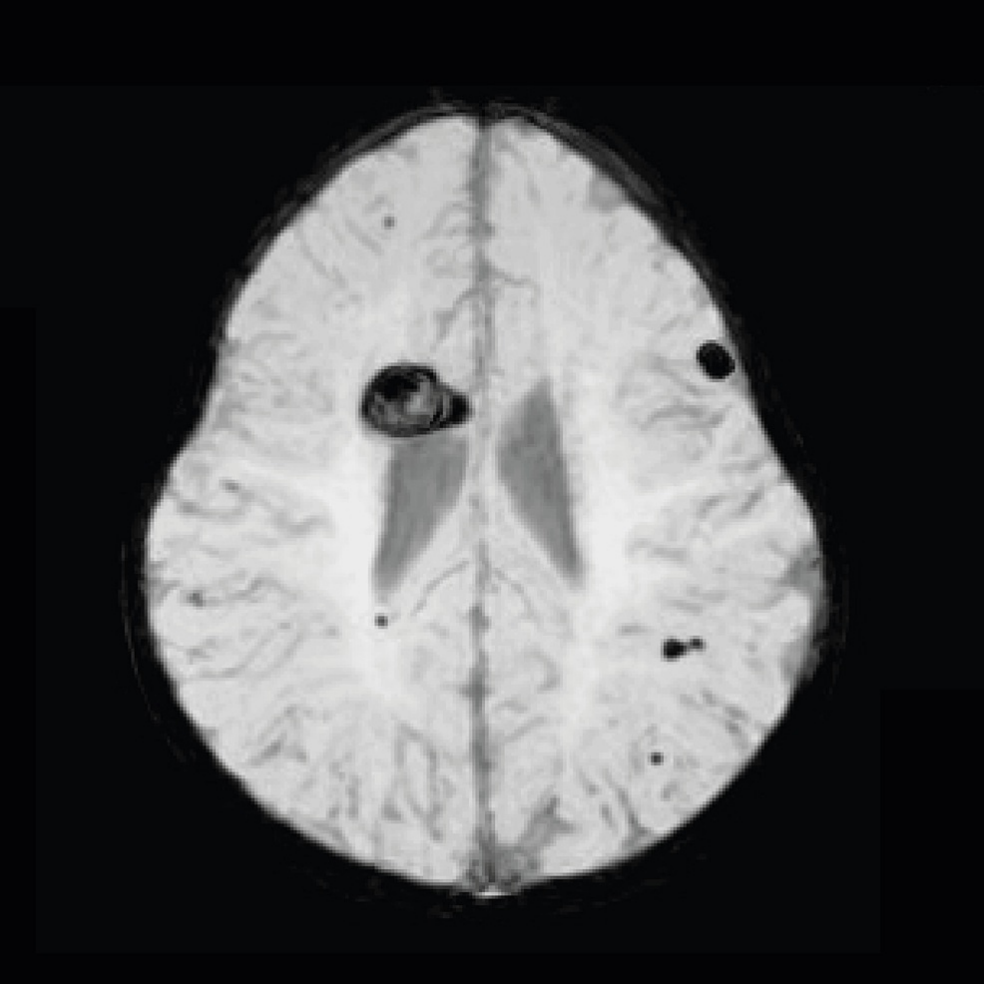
Susceptibility-weighted image from brain MRI. Multiple cavernous malformations are seen in our patient. Largest mass is in the roof of the left ventricle. Cavernous malformation is also seen in the brain, distributed in both hemispheres of the five-month-old patient.
